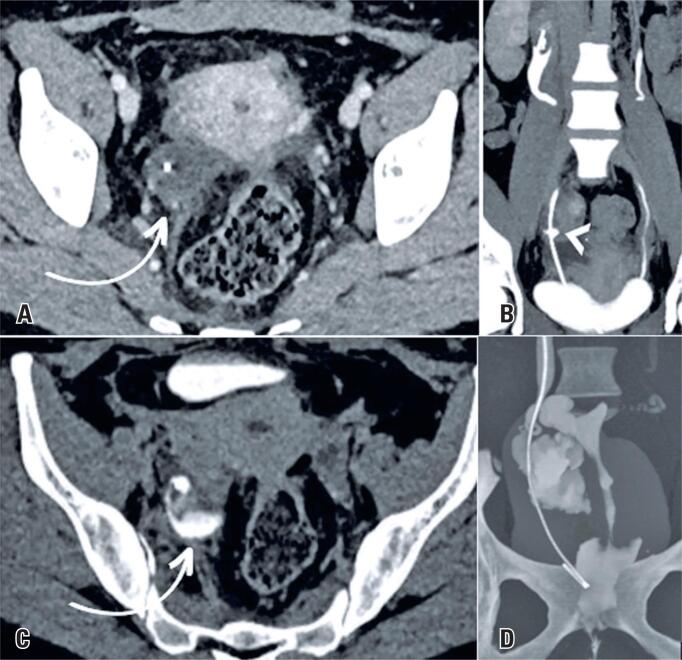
Axial (A and C) and coronal (B) contrast-enhanced CT scans showing a hypodense collection on the right of the pelvic excavation (white arrows), with a component in the adnexal region and near the lower third of the ureter, identifying extravasation of contrast in the excretory phase in the lower third of the ureter (arrowhead) that fills the mentioned collection, suggesting urinoma (C). Coronal MIP contrast-enhanced CT scan (D) showing extravasation of contrast into the collection and into the right uterine tube, suggesting a uretero-fallopian fistula, with consequent passage of contrast into the uterine cavity and vagina
